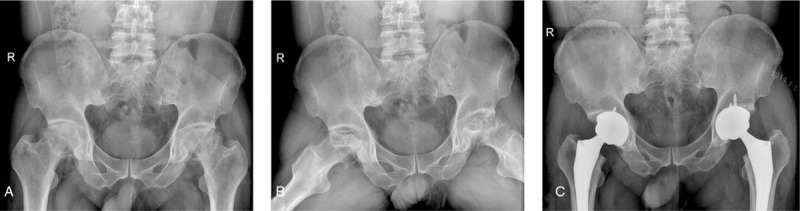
X-ray examination of pelvis (A and B) before and (C) after operation.
